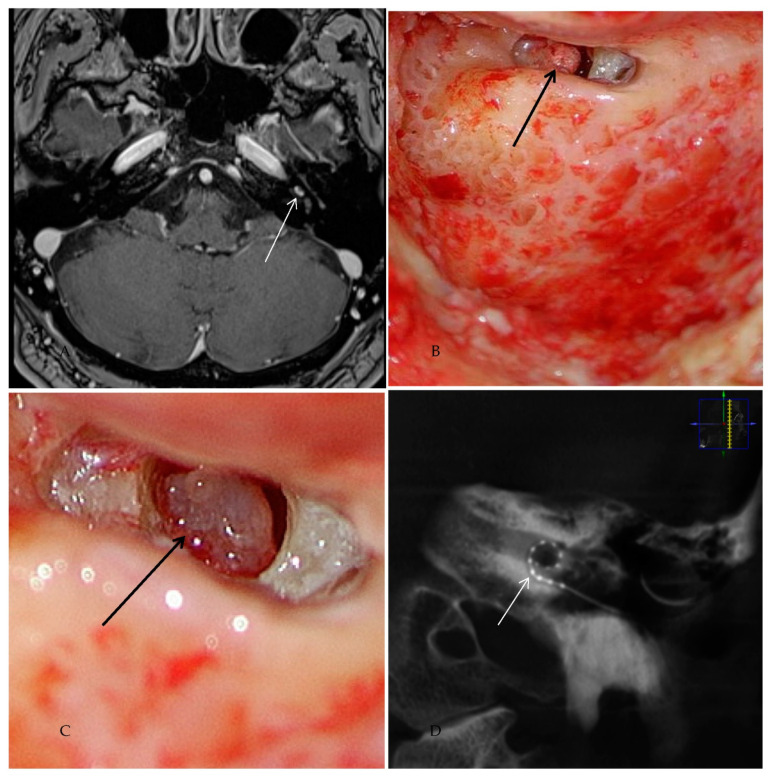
Case #3: ILS of the middle cochlear turn (A) MR image T1 VIBE with i.v. gadolinium: the white arrow points to the intracochlear schwannoma in the middle turn. (B) Intraoperative image of the subtotal cochleoectomy exposing the schwannoma (black arrow) in the middle turn. (C) Magnified image of the intracochlear schwannoma in the middle turn (black arrow). (D) Postoperative ConeBeam CT of the CI electrode (white arrow) in the cochlea.
